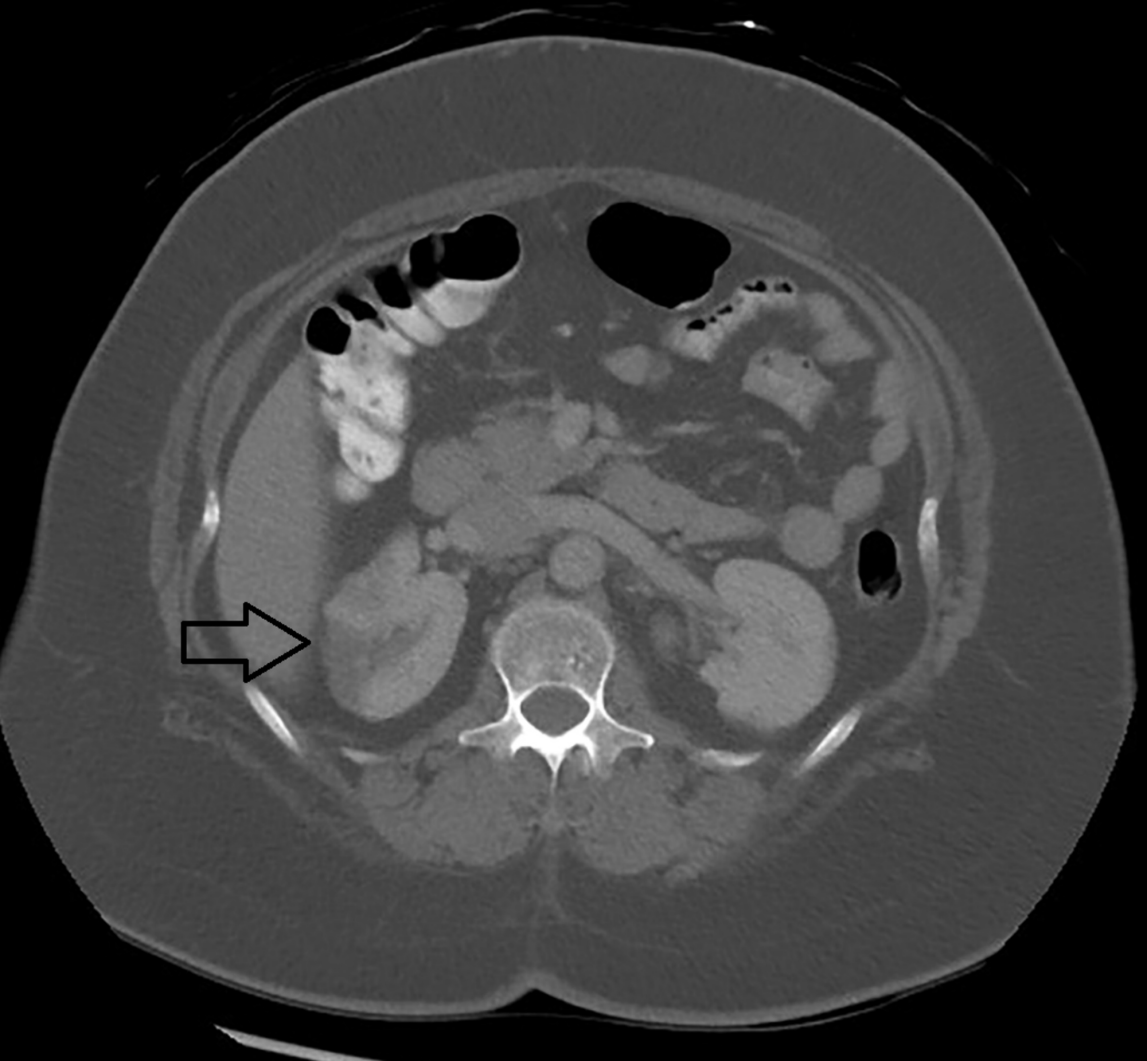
Computed tomography of the abdomen showing an infarct area measuring 42 mm x 22 mm x 27 mm in the upper pole ( dark arrow) and additional subtle hypodensity in the anterior aspect of the midpole of the right kidney. Bilateral renal scarring is present.
